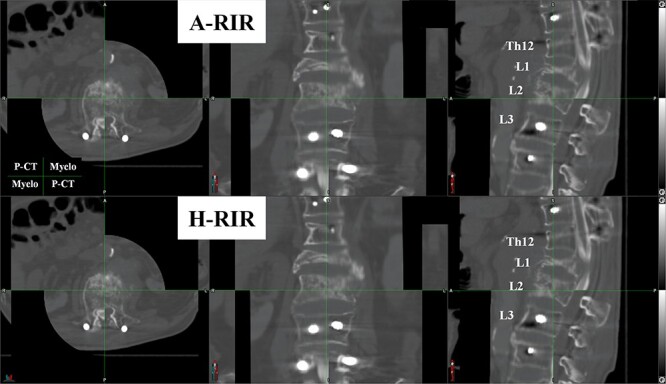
An example of the blind review comparing two registration results. Each patient’s registrations were displayed on the horizontal split screen with automatically shuffled. Three evaluators are prompted to choose the registration they would like to use clinically. The figure is shown on a checkerboard display (the planning CT: the upper left and lower right parts, the CT-myelogram: the upper right and lower left). The evaluators can review all the slices of CT images. A-RIR: automated rigid image registration, H-RIR: human rigid image registration, P-CT: planning CT, Myelo: CT-myelogram.
